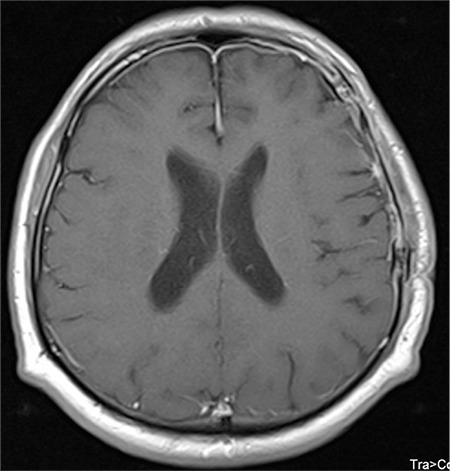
Postoperative cranial magnetic resonance imaging.
